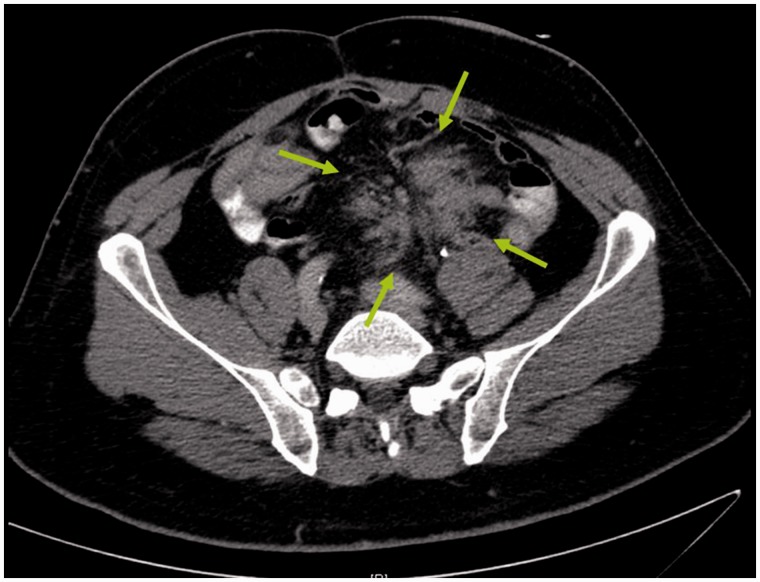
Image from a computed tomography of a desmoid tumor in a patient with familial adenomatous polyposis. The tumor is marked by the arrows. The tumor has developed many years after staged total proctocolectomy with end ileostomy. A surgical attempt to resect the tumor was undertaken, without success.
